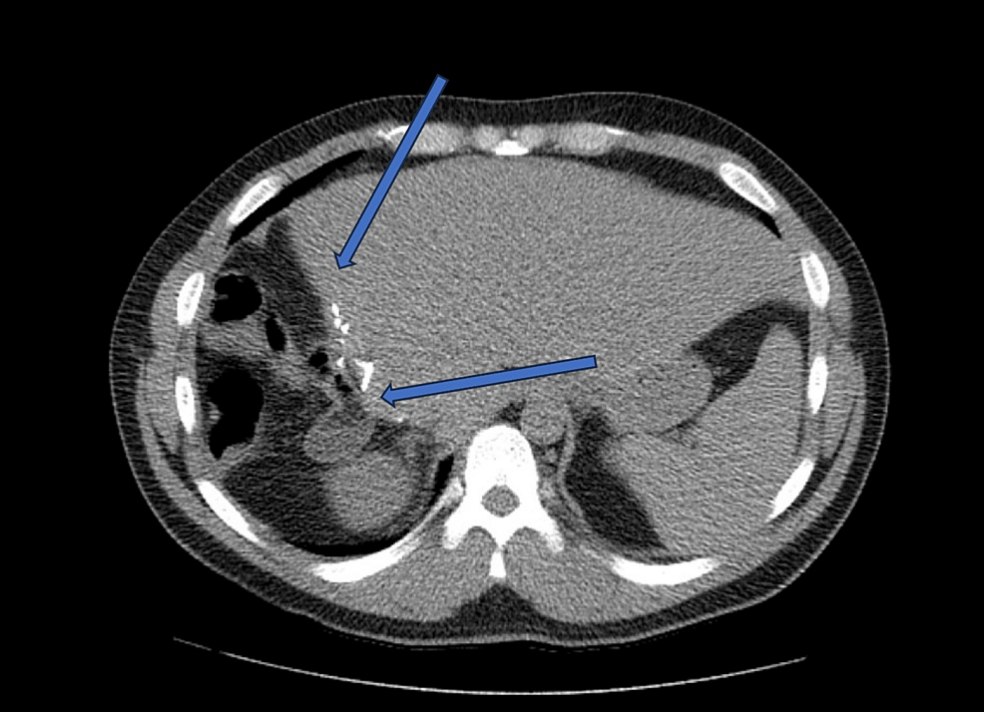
CT scan of abdomen and pelvis with IV contrast Six-month follow-up CT scan demonstrated a stable postoperative condition, with no enhanced hepatic lesions observed.
